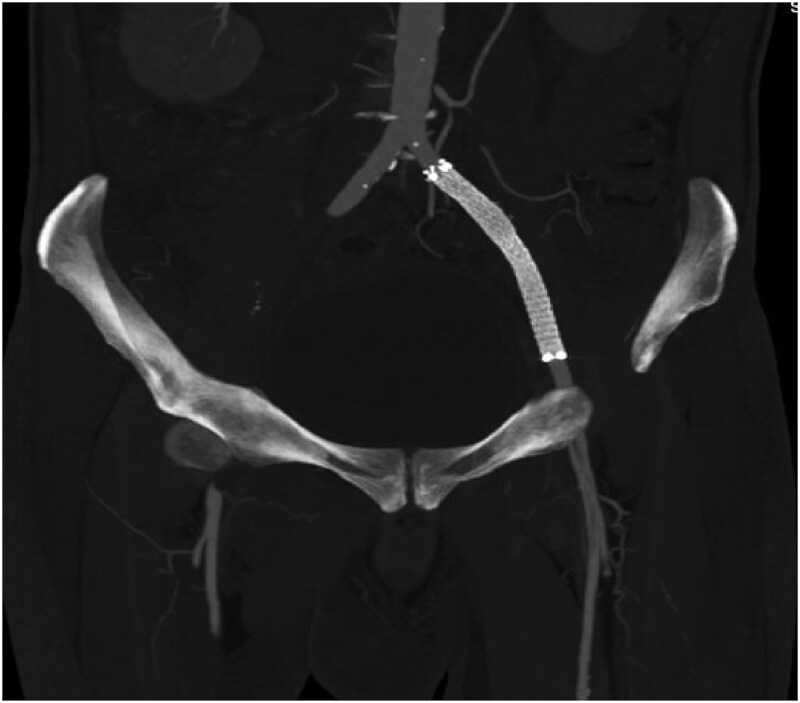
Follow-up Computed tomography angiography at three months shows the patent-covered stent in the left external iliac artery with good distal runoff.
